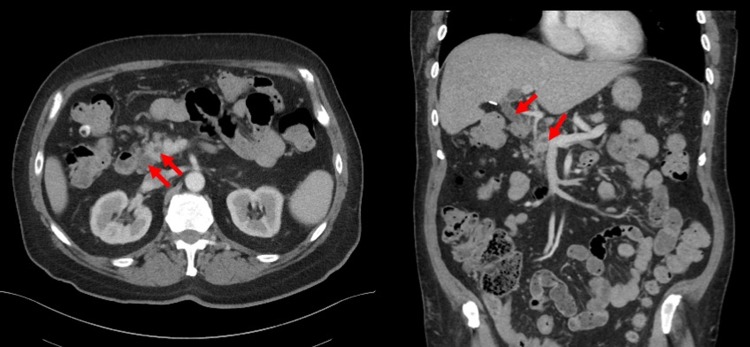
Relevant imaging for case 2: select contrast-enhanced CT imaging demonstrating a double duct sign (*red arrow*) where both the common bile duct and main pancreatic duct are simultaneously visualized and dilated.
